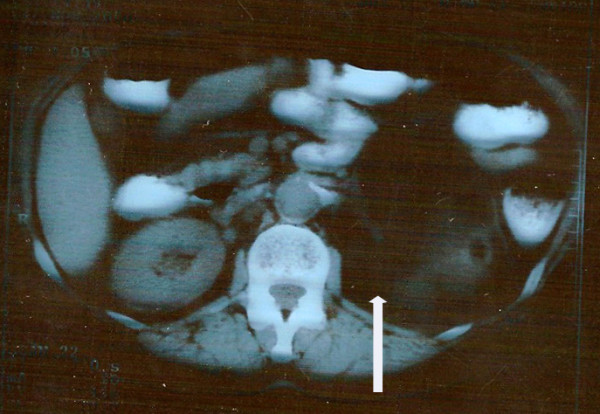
Computed tomography scan demonstrating a well circumscribed tumor (arrow) in the left retroperitoneal space with adipose tissue density (Hounsfield units = 100) after oral administration of contrast agent.
